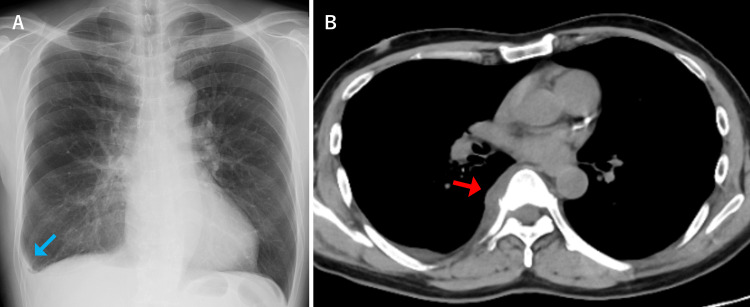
Chest radiography and plain chest CT images on initial examination CT: computed tomography (A) Chest radiography shows blunting of the right costophrenic angle, suggesting a small amount of pleural effusion (blue arrow). (B) Chest CT shows mild soft tissue density on the right side of the T8-9 vertebral bodies (red arrow)
